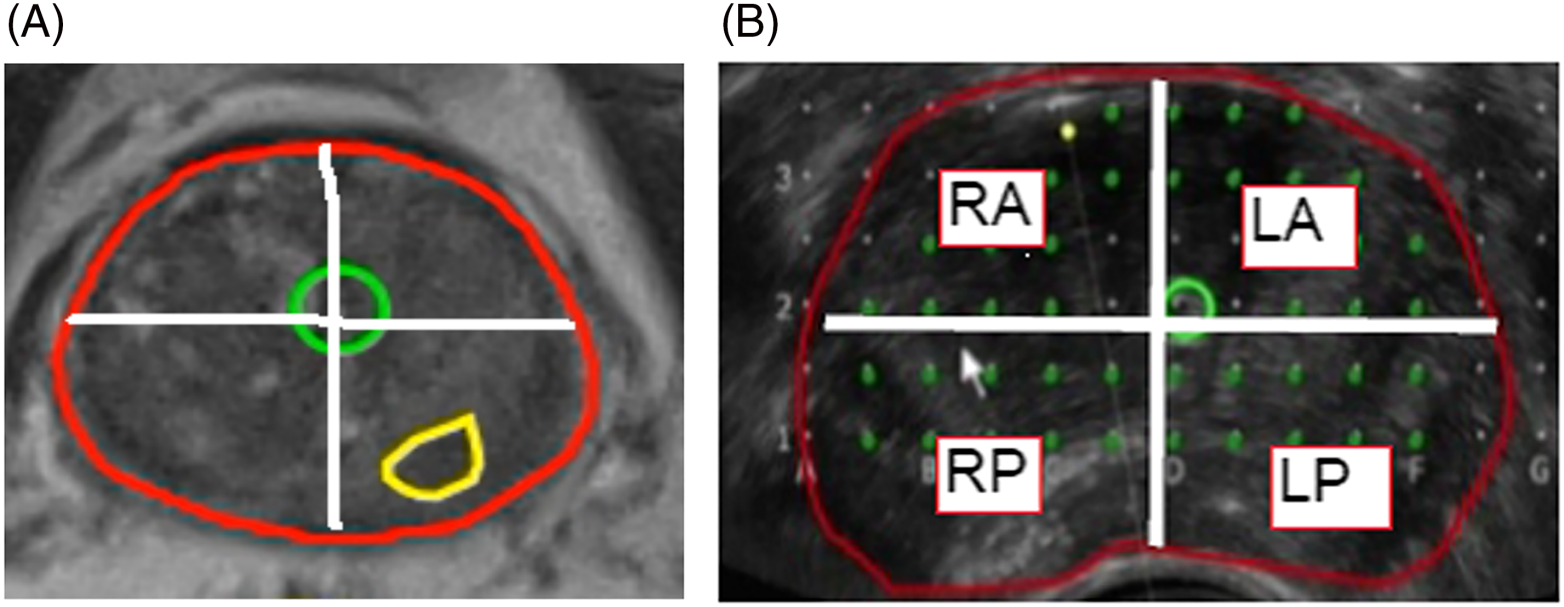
(A) T2 sequence of multiparametric magnetic resonance imaging (mpMRI) with prostate divided into 4 sectors with urethra (green circle) as axis. Region circled in yellow represents PI‐RADs 4 lesion. (B) Four sectors (quadrants) from axial ultrasound prostate image. Red line represents circumference of prostate and green circle urethra. Green dots are template puncture sites. RA—right anterior, LA—left anterior, RP—right posterior, LP—left posterior. Proprietary software programme not available for clinical use
